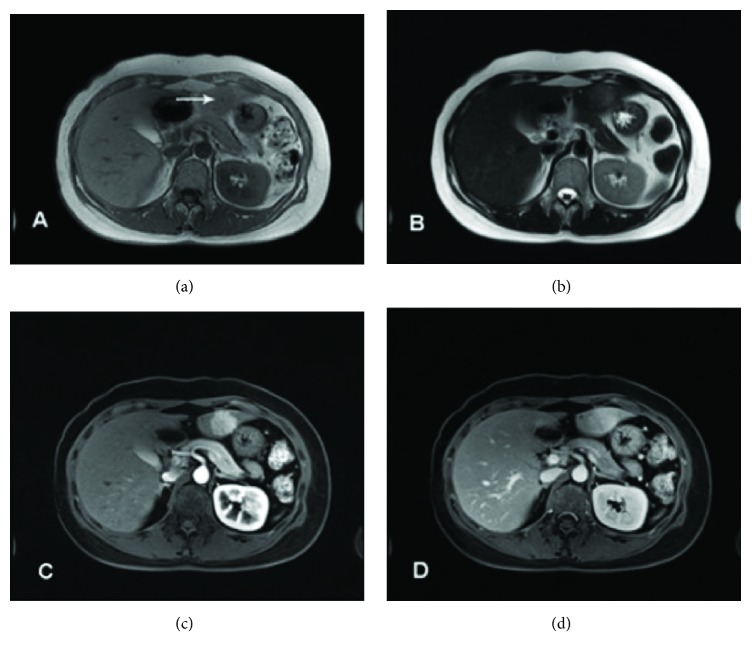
Abdominal magnetic resonance imaging (MRI) findings: (a) T1-weighted image shows that the lesion is slightly hypointense with the central scar appears more significant hypointense (arrow); (b) T2-weighted images shows the same lesion as slightly hyperintense; (c) after enhancement, the signal of the lesion is markedly increased and appears hyperintense during the arterial phase except for the central area in fat-saturated T1-weighted image; (d) in the portal venous phase, the lesion is slightly hyperintense compared to the normal liver tissue and the central scar is poorly recognized.
